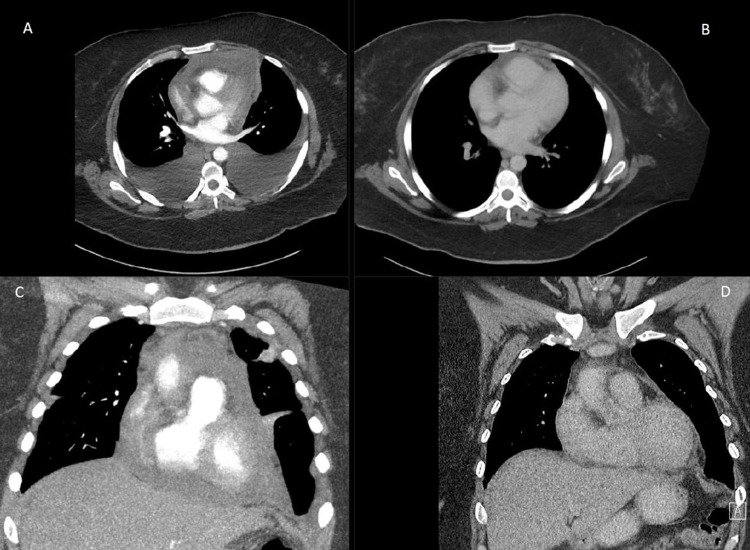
Computed tomography chest with intravenous contrast prior to initiation of Cladribine (left column, images A & C) compared to contrasted CT chest imaging after six cycles of cladribine (right column, images B & D), demonstrating complete radiographic resolution of pericardial and pleural effusions.
